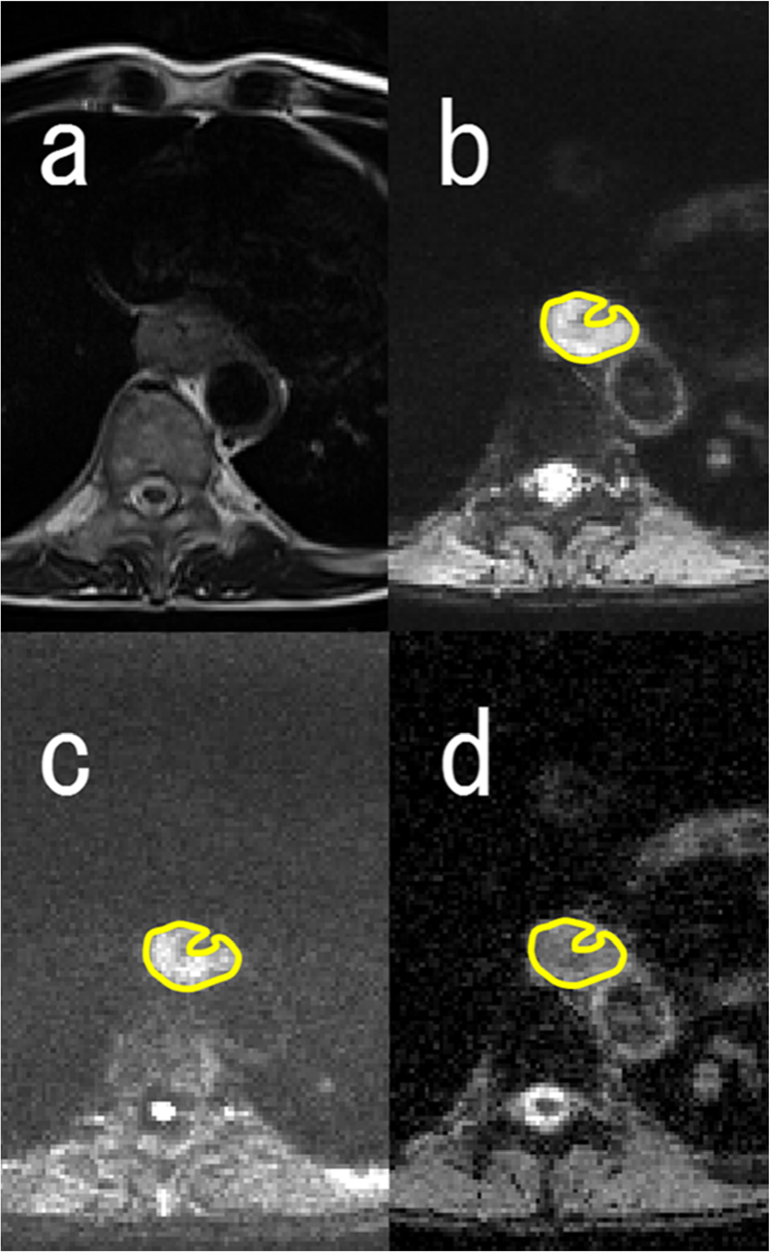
Axial MR images. a T2-weighted image, b diffusion-weighted image (DWI) with *b* = 50 s/mm^2^, c DWI with *b* = 800 s/mm^2^ and d apparent diffusion coefficient (ADC) map of a 62-year-old man with oesophageal squamous cell carcinoma. Region of interest (ROI) placement on DWI with *b* = 50 s/mm^2^ (b) was performed by referring to the outline of the tumour that showed intermediate to high intensity with oesophageal wall thickening on T2-weighted images (a). Each ROI was placed to cover the entire primary lesion without the lumen and was transferred to the corresponding ADC map (d)
